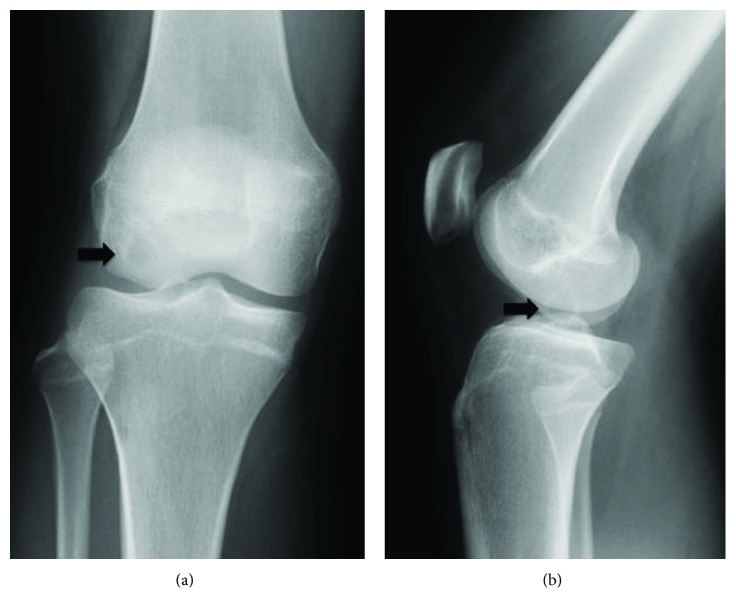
(a, b) A radiograph shows an epiphyseal osteolytic lesion in the end of the right femur (arrow).
